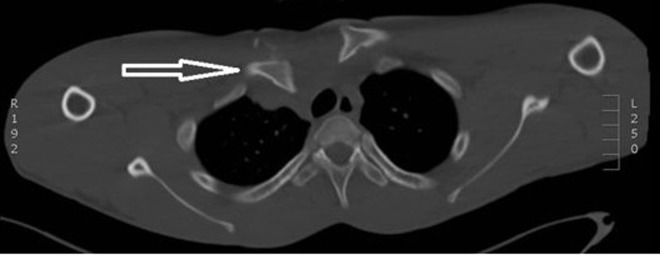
computed tomography scan view of the sternoclavicular dislocation
